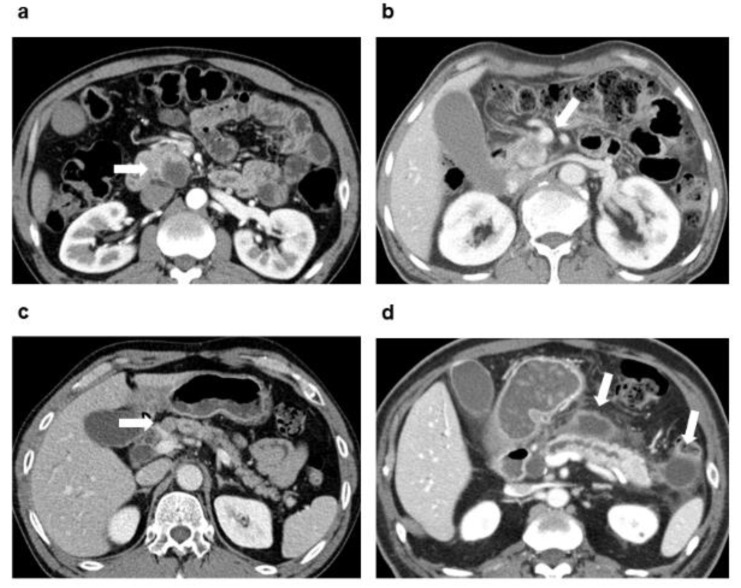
Four preoperative imaging characteristics included in the nomogram to predict one- and five-year DFS and OS in patients with pancreatic head cancer who underwent curative-intent surgery without neoadjuvant therapy. Presence of (a) necrosis (centrally located cystic change of the tumor with irregular internal margin), (b) worrisome finding for invasion of PV or SMV according to the NCCN guidelines, (c) suspected metastatic regional lymph node (short diameter > 8 mm, round, irregular or necrotic change), and (d) associated pancreatitis or pseudocyst on CT. DFS, disease-free survival; OS, overall survival; PV, portal vein; SMV, superior mesenteric vein; NCCN, National Comprehensive Cancer Network; CT, computed tomography.
